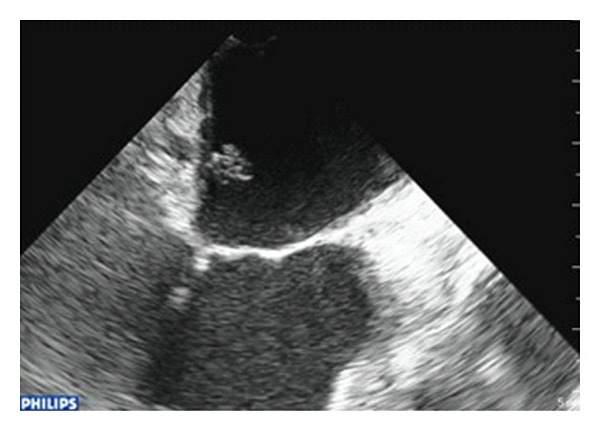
Transesophageal image of the left atrial mass.
